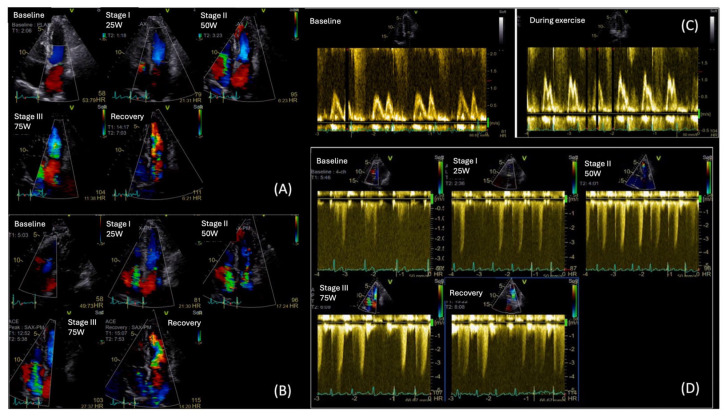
Diastolic Stress Echo in a T2DM patient with exertional dyspnea: (A) Color Flow Doppler (CFD) showing mild Mitral Regurgitation throughout the test, (B) CFD of Tricuspid Regurgitation (TR) augmenting during exercise, (C) Mitral inflow velocities at rest (left image) and during exercise (right image). We can observe a change in mitral inflow pattern, towards type II diastolic dysfunction, (D) Maximum TR velocity increase during exercise, from 2.5 m/s at rest to 4 m/s during exercise at low workload. (Original images from authors’ institutions).
